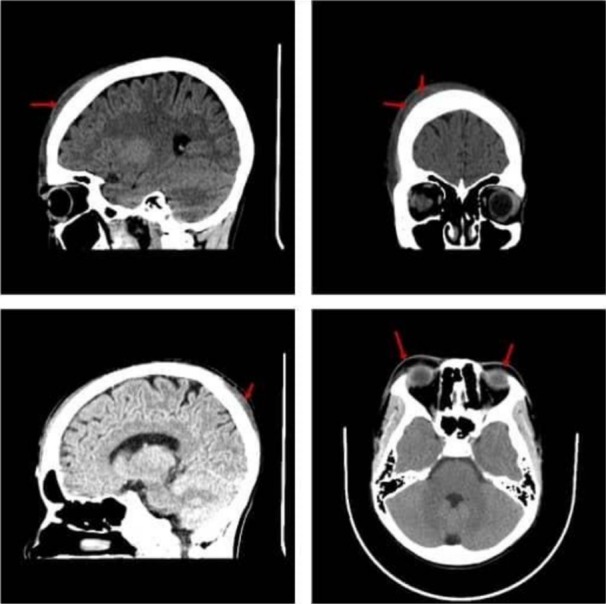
Non‐contrast enhanced head CT scan showing subgaleal hematoma (red arrows) of the right frontal and occipital scalp regions, extensions to the right temporal and bilateral upper eyelids.
